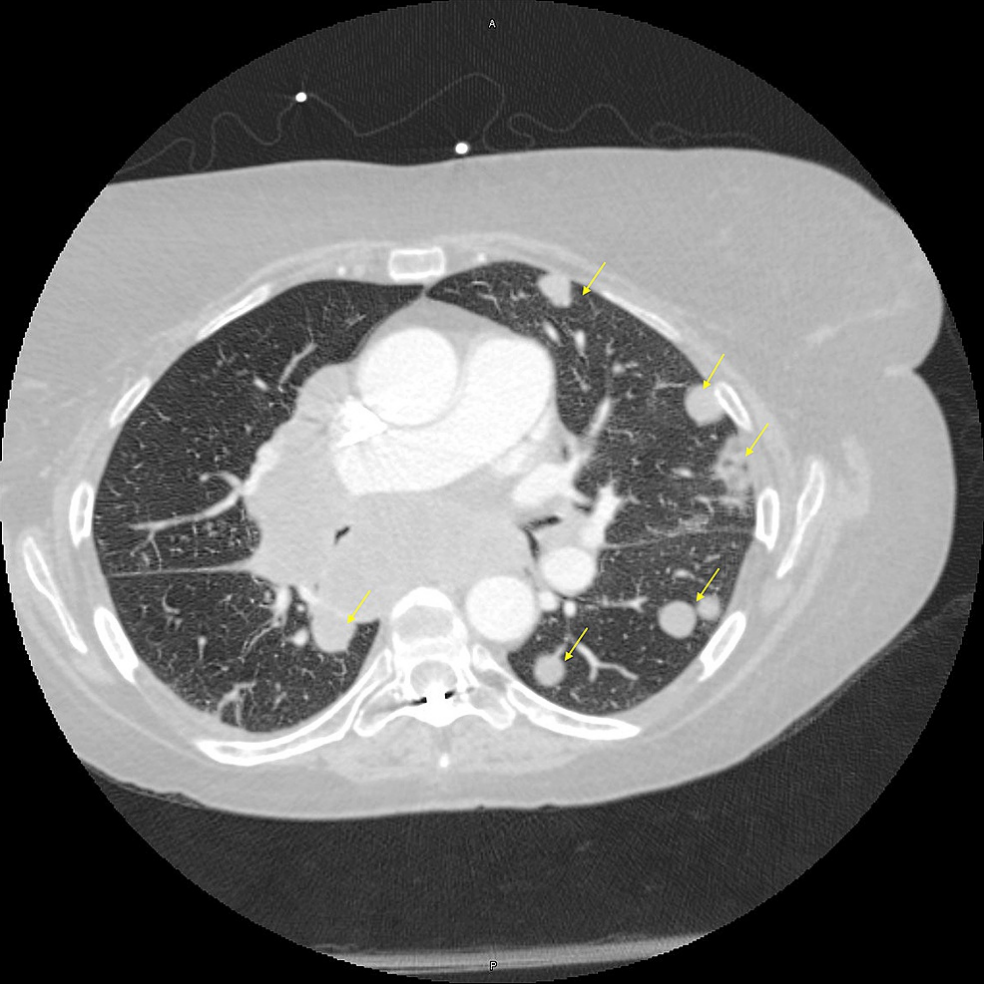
Axial section of the CT pulmonary angiogram. Multiple rounded pulmonary angiosarcoma metastatic lesions. Yellow arrows: Multiple pulmonary nodules
